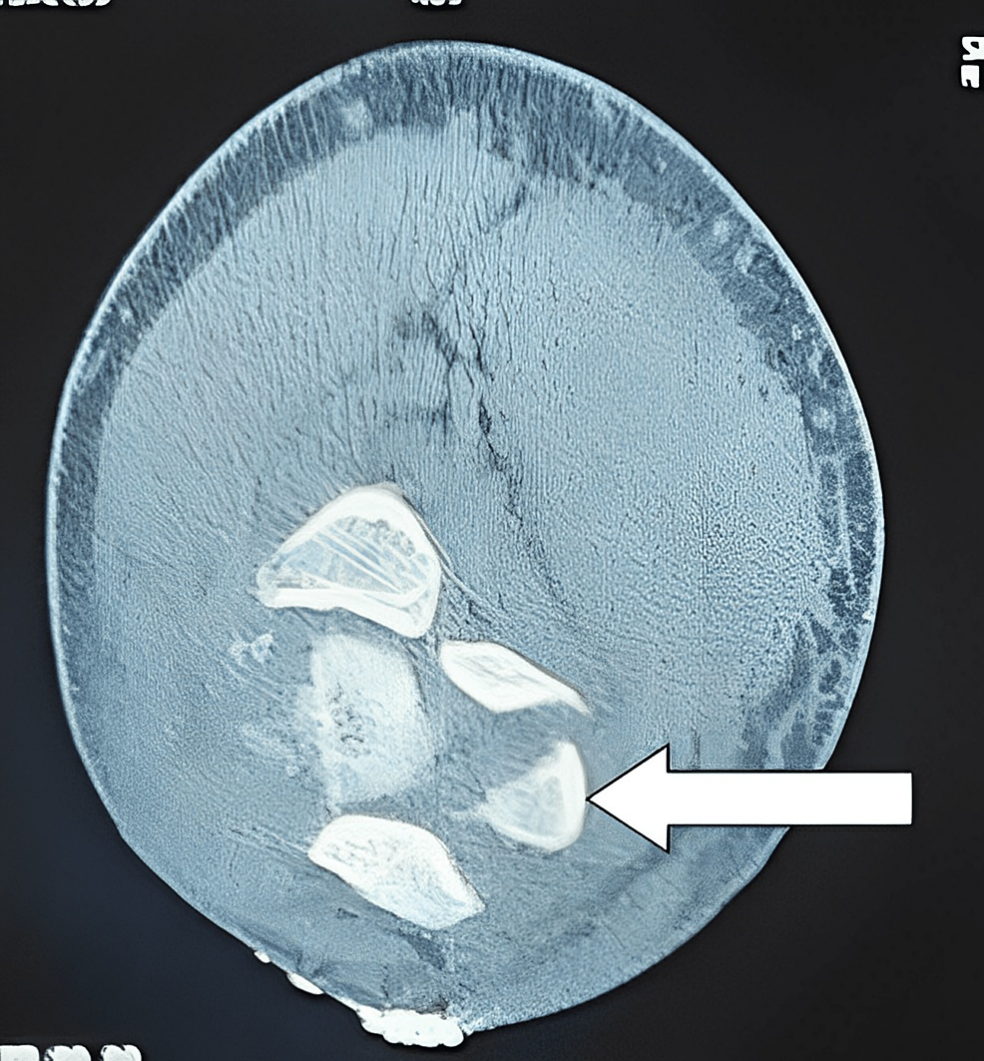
CT of left elbow (axial image) showing fractured fragment (white arrow) into the joint.
